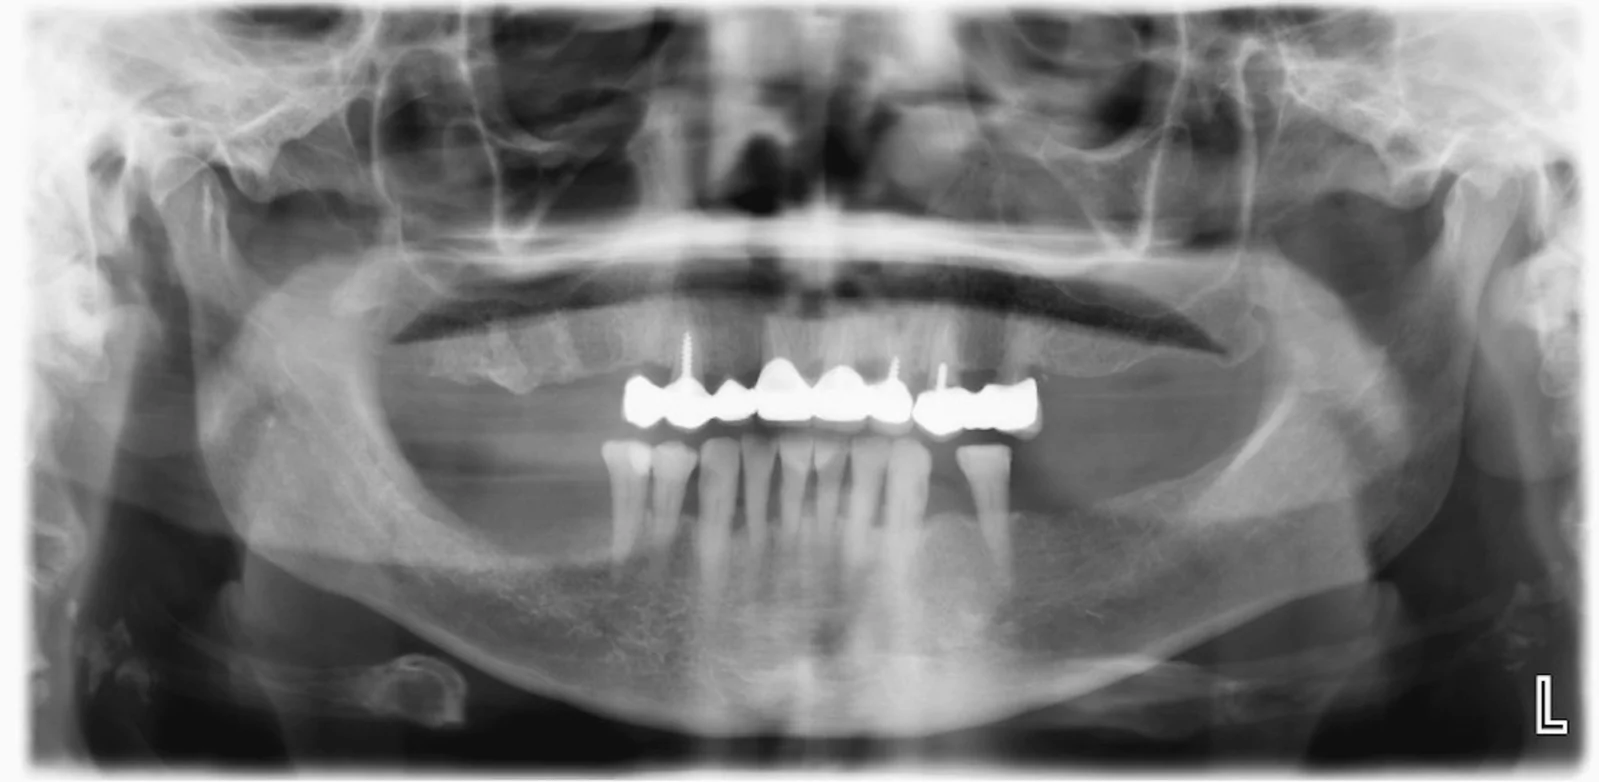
A panoramic radiograph taken on May 2024 depicting a subtle radiolucency of the alveolar crest in the right mandibular molar region
